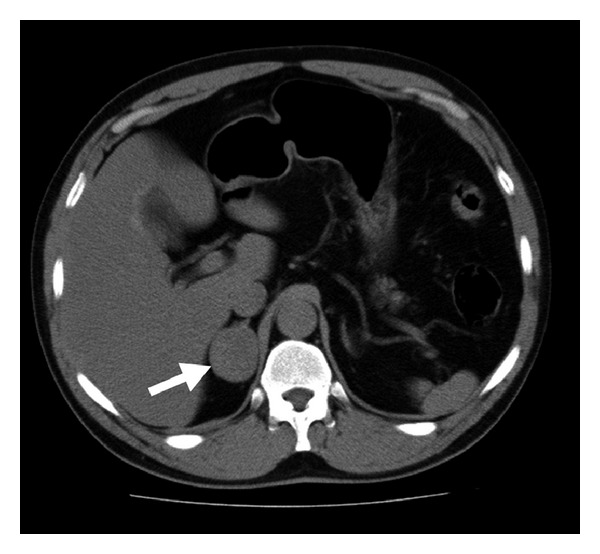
Computed tomography showing a mass in the right adrenal gland (arrow).
